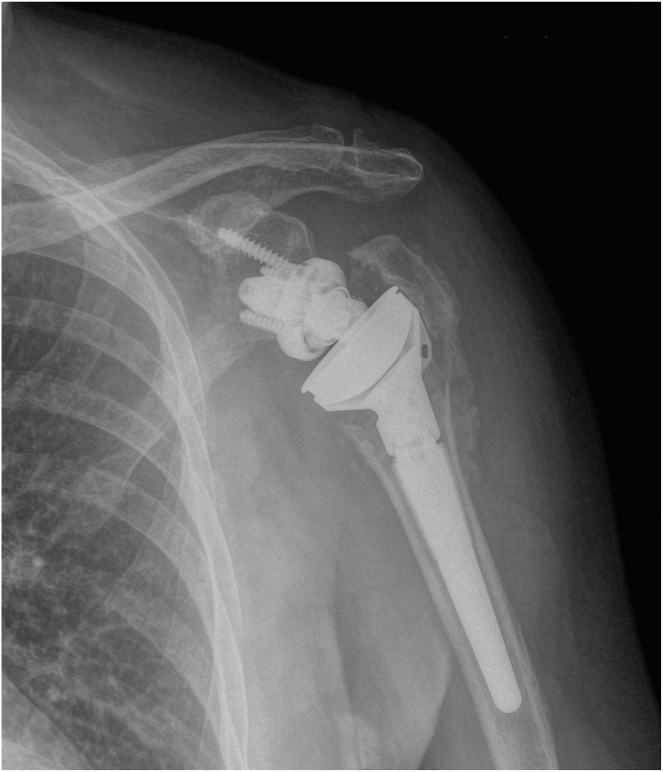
Follow-up radiograph of *Left* shoulder at 24 months postoperatively. In this 82-year-old patient, a malunion of the GT is observed without any migration. *GT*, greater tuberosity.
